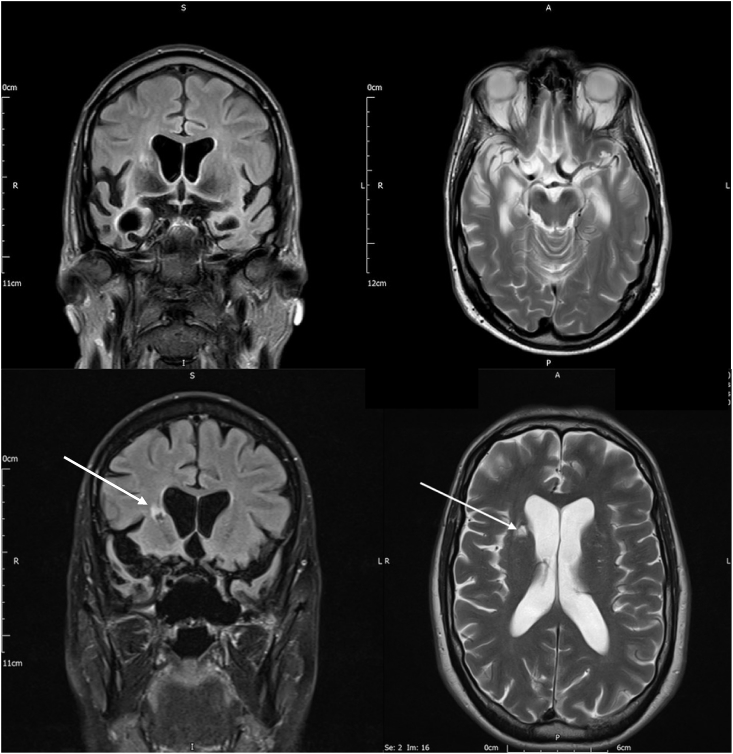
Magnetic Resonance Imaging scan of patient SA. This was reported as showing bilateral anterior temporal lobe atrophy, worse on the right than the left. A small, old lacunar infarct in the right putamen was also noted (indicated by arrows).
